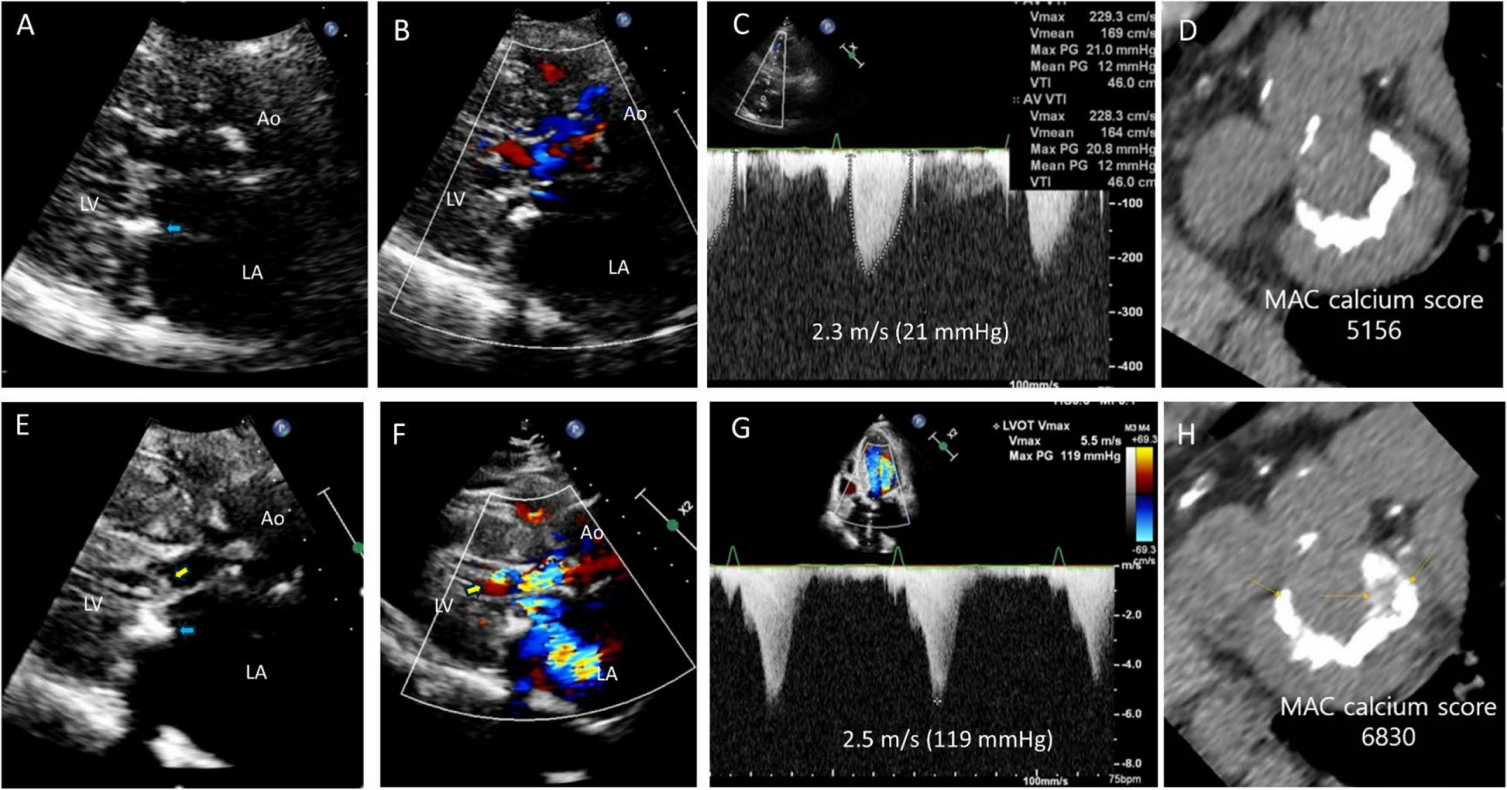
A representative case showing the contribution of progression of mitral annular calcification to left ventricular outflow tract obstruction. A 67-year-old woman with no history of hypertrophic cardiomyopathy showed (A, B) mitral annular calcification and hypertrophied papillary muscle without (C) significant left ventricular outflow tract (LVOT) obstruction. E–G Four years later, severe flow acceleration and LVOT obstruction were documented. D, H Computed tomography clearly showed the progression of mitral annular calcification with increased calcification extent and calcium score
